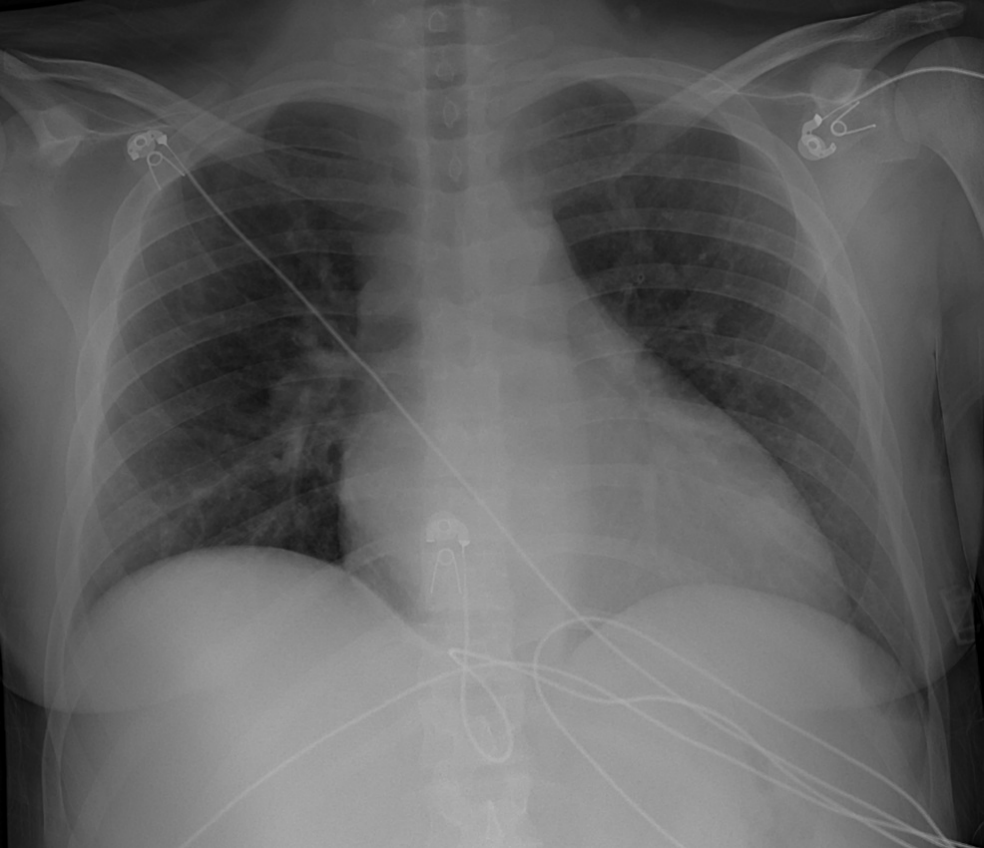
Chest X-ray showed clear lung fields with no signs of consolidation
